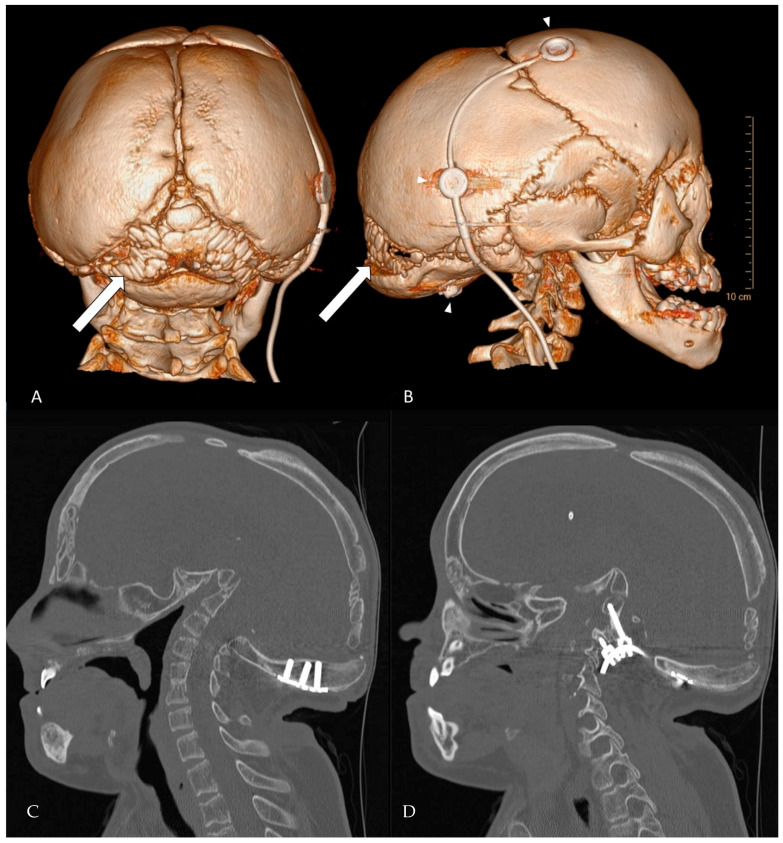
CT-rendering of Wormian bones at the age of 18 years. (A) posterior view of the skull. (B) lateral view. The arrows point to multiple intrasutural bone fragments arising in the lambdoid suture, a fragment can be seen in the coronal suture and pterion ossicles are present. The upper two arrowheads depict the VP-shunt system, the lower arrowhead points to a screwhead of the occipital instrumentation. (C,D) Complementary 2D bone-window CT slices (sagittal plane) detailing instrumentation the position and anatomy that are difficult to appreciate on 3D views. (C) Mid-sagittal slice showing the relationship of the occipital plate/screws to the foramen magnum and the cranio-cervical junction. (D) Left-parasagittal slice highlighting the occipito-cervical instrumentation and screw trajectory.
